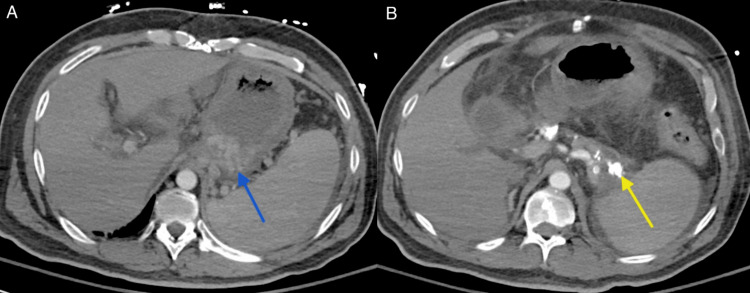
CT abdomen/pelvis with IV contrast A: Diffusely thick-walled and distended stomach with gastric varices (blue arrow) and splenomegaly. B: Extensive pancreatic calcification (yellow arrow).
